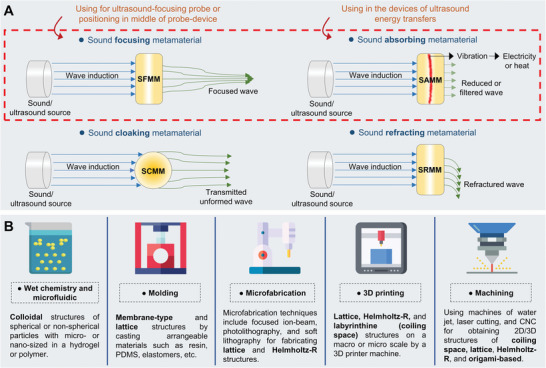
A) The classification of UMMs with their functions on sound/ultrasound wave manipulating. B) The categorization of fabrication techniques for UMMs.
